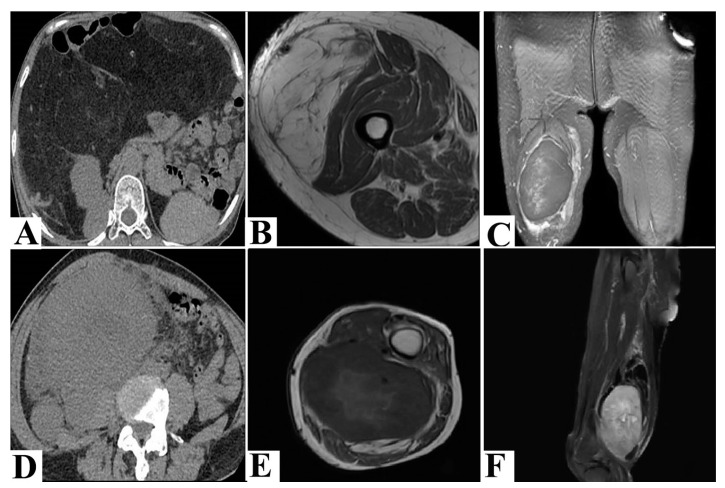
(A) Computed tomography image shows a well-differentiated liposarcoma located in the upper abdomen, with well-defined borders, fat-like content, and some septa over 2 mm pushing the surrounding tissues. Adapted from Yang, T. et al. (2025) [26] and licensed under CC BY 4.0. (B) Well-differentiated liposarcoma located at the vastus lateralis level in axial and T1 sequences with hyperintensity of the lesion and hypointensity of the septa. Adapted from Natella, R. et al. (2023) [22] and licensed under CC BY 4.0. (C) Well-differentiated liposarcoma on T2-weighted fat-suppressed image reveals a well-defined, low-signal adipose tissue mass with thickened septa in the right thigh. Adapted from Zhang T. et al. (2024) [25] and licensed under CC BY NC 4.0. (D) A dedifferentiated liposarcoma located in the right lower abdomen, with margins imprecisely delimited from the surrounding tissues, and solid content invading the right psoas major muscle. Adapted from Yang, T. et al. (2025) [26] and licensed under CC BY 4.0. (E) Dedifferentiated liposarcoma on T1-weighted images presents as an indistinct adipose tissue mass in the left popliteal fossa with low signal non-fatty areas and heterogeneous areas. Adapted from Zhang T. et al. (2024) [25] and licensed under CC BY NC 4.0. (F) Liposarcoma on T2-weighted images with fat suppression shows low-signal suppressed adipose areas and high-signal non-fatty areas, as well as thick septations in the adipose areas. Adapted from Zhang T. et al. (2024) [25] and licensed under CC BY NC 4.0.
